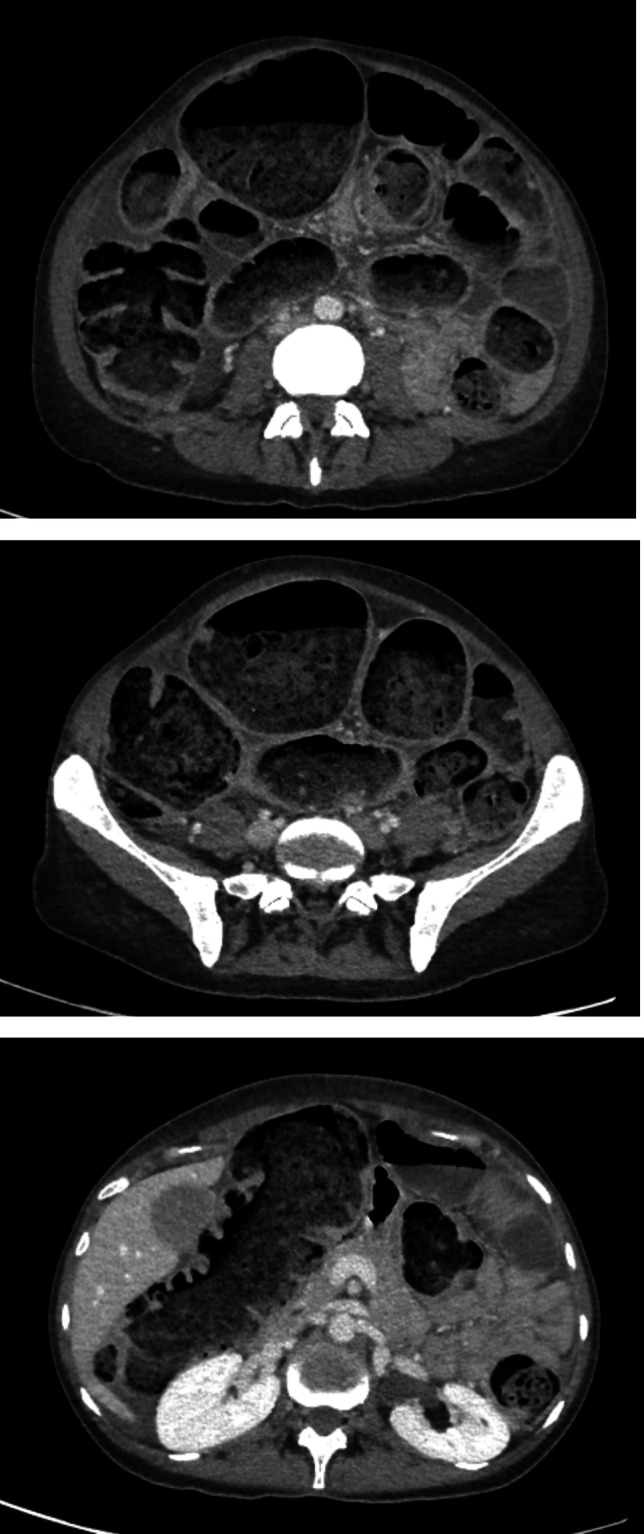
CT scan from patient 3 revealed a pseudo-occlusive syndrome
